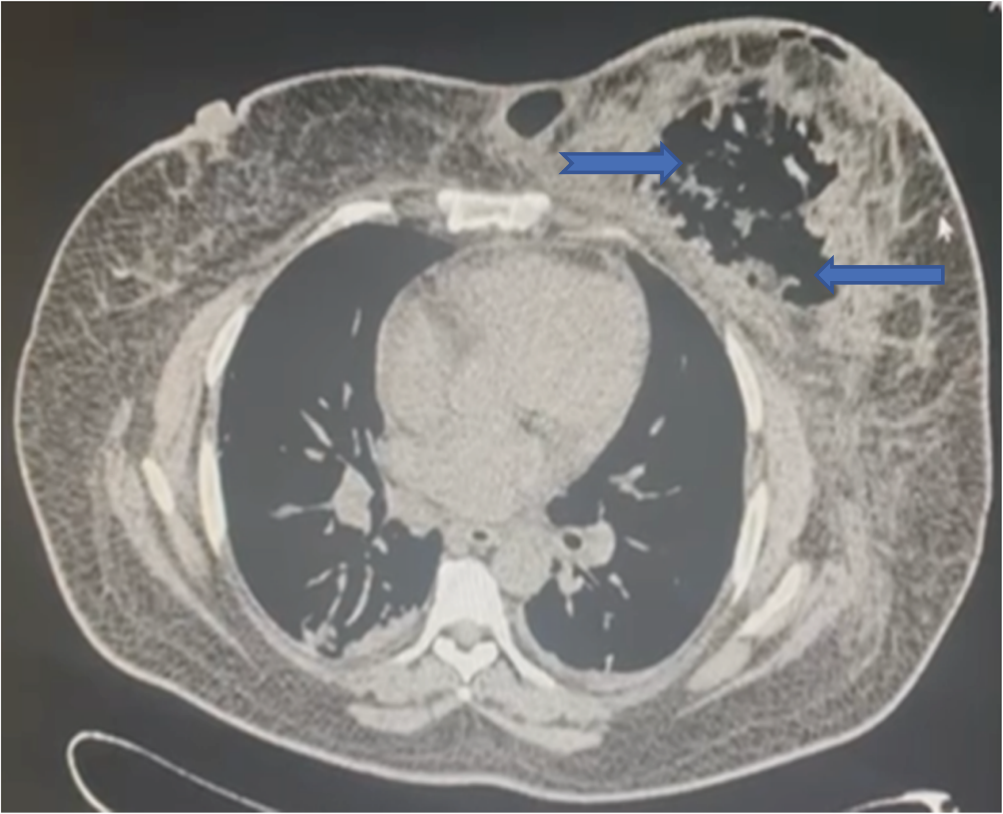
CT scan of the chest showing extensive gas within the left breast (see blue arrows).
